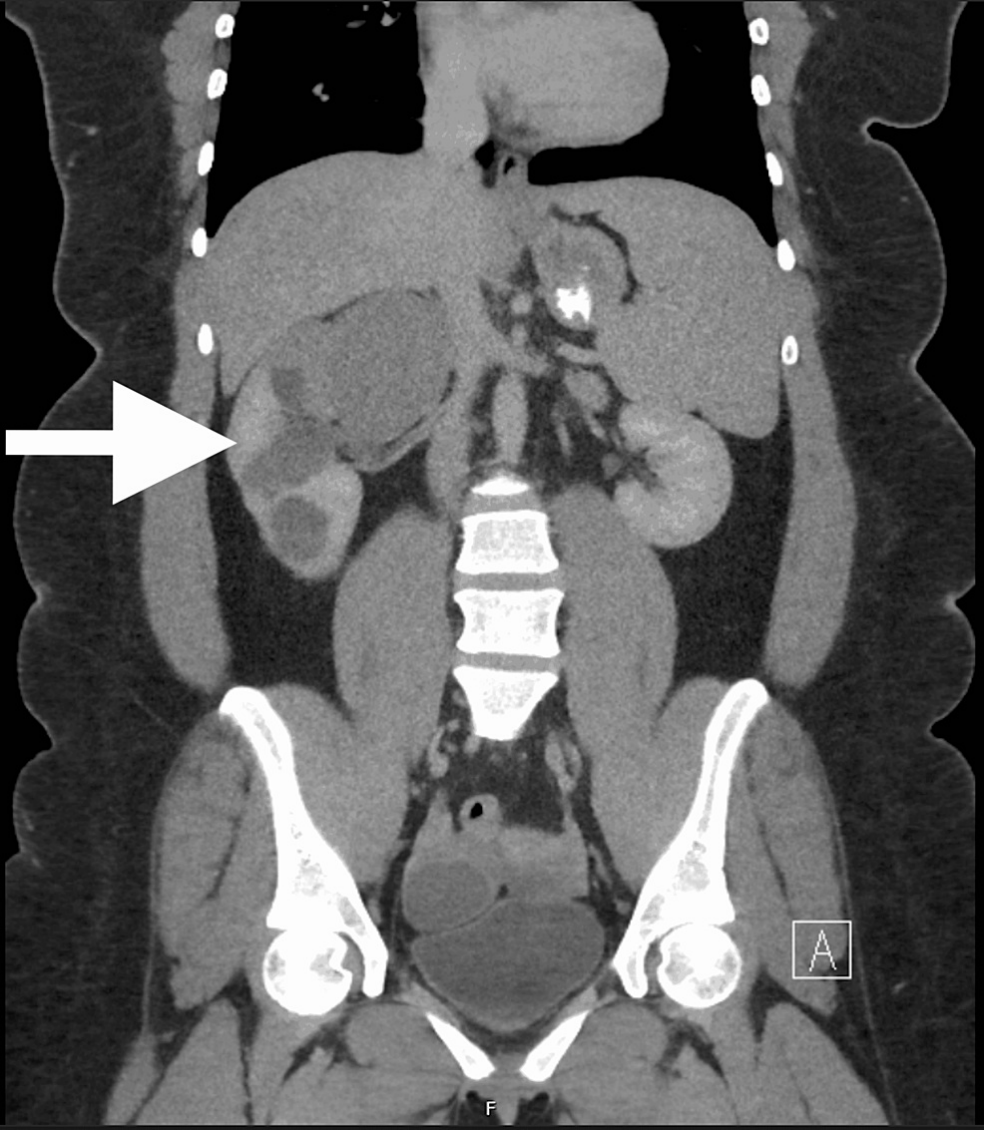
Abdominal computed tomography scan demonstrating multiple cortical and renal sinus cysts in the right kidney. No involvement of the left kidney was identified.
